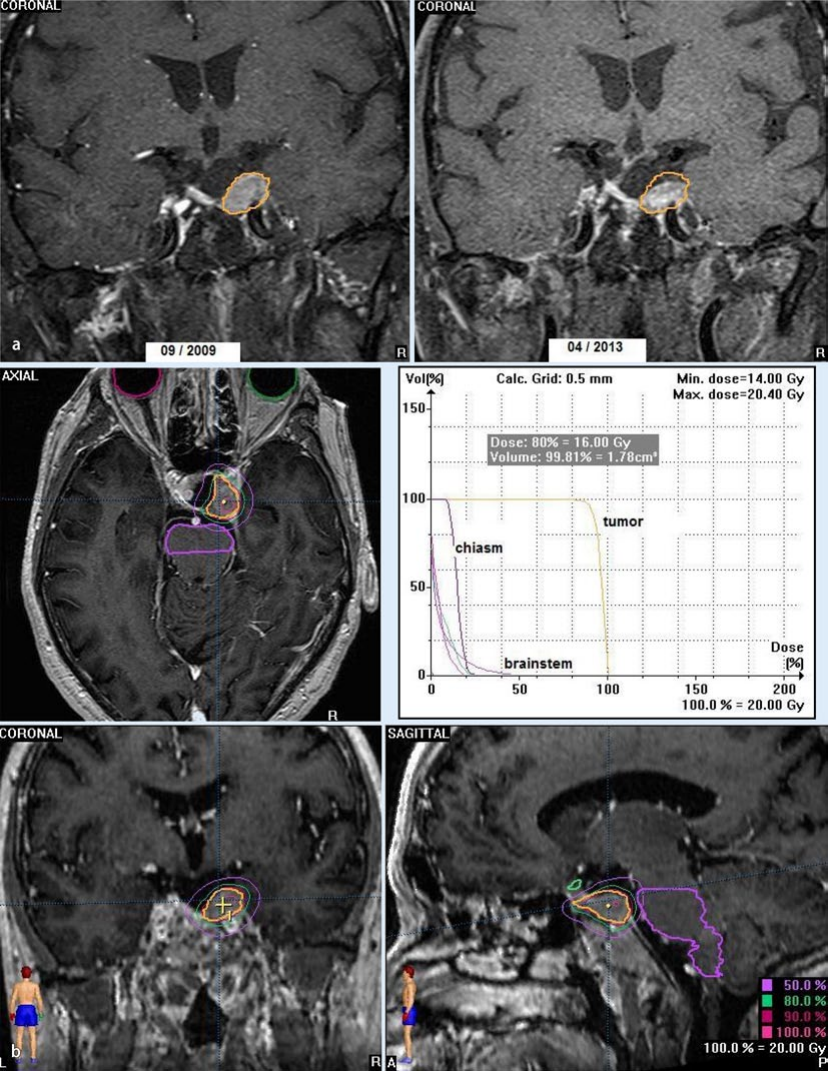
a, b MRI of patient (male, 72 years) with tumor progression after 2 transsphenoidal surgeries (the last in 2005), SRS with 20 Gy in March 2009 (CTV 1.88 ccm). At 4-year follow-up, tumor regression, no visual disorder, no hypopituitarism
